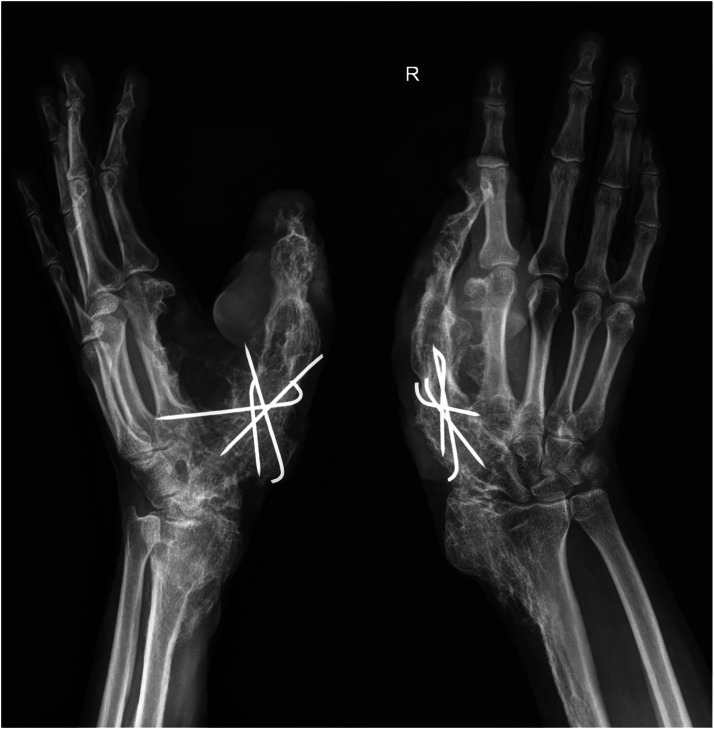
X-ray pictures of the patient’s right hand 2 months after the first surgical stage, before the K-wires removal.
